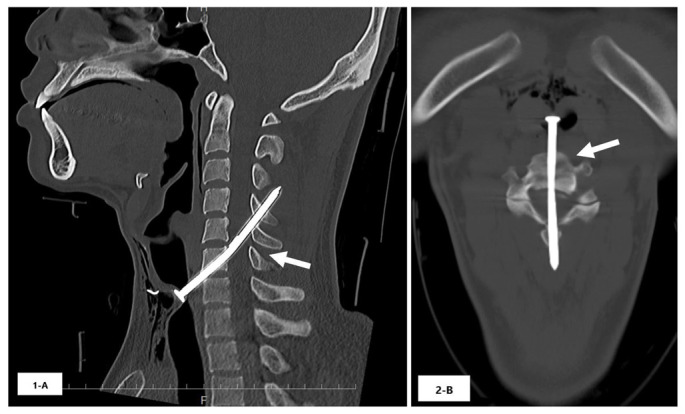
Cervical computed tomography showing a 9-centimeter nail penetrating the trachea, anterior vertebral body, and spinal cord (arrows).
